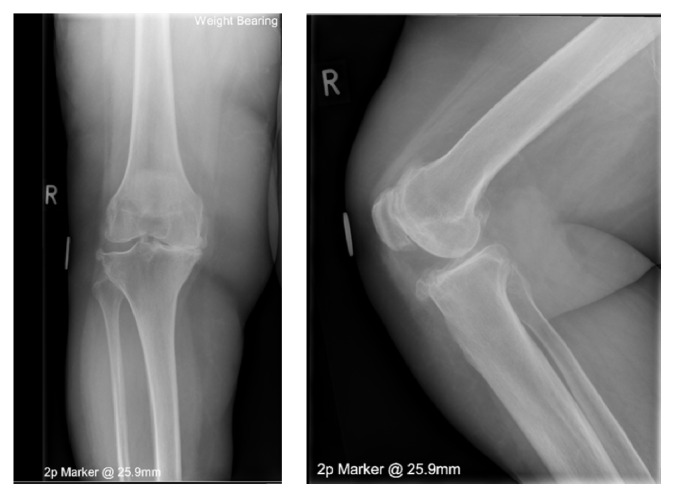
Preoperative weight-bearing AP and lateral radiographs clearly showing tricompartmental knee OA (K-L Grade IV).
